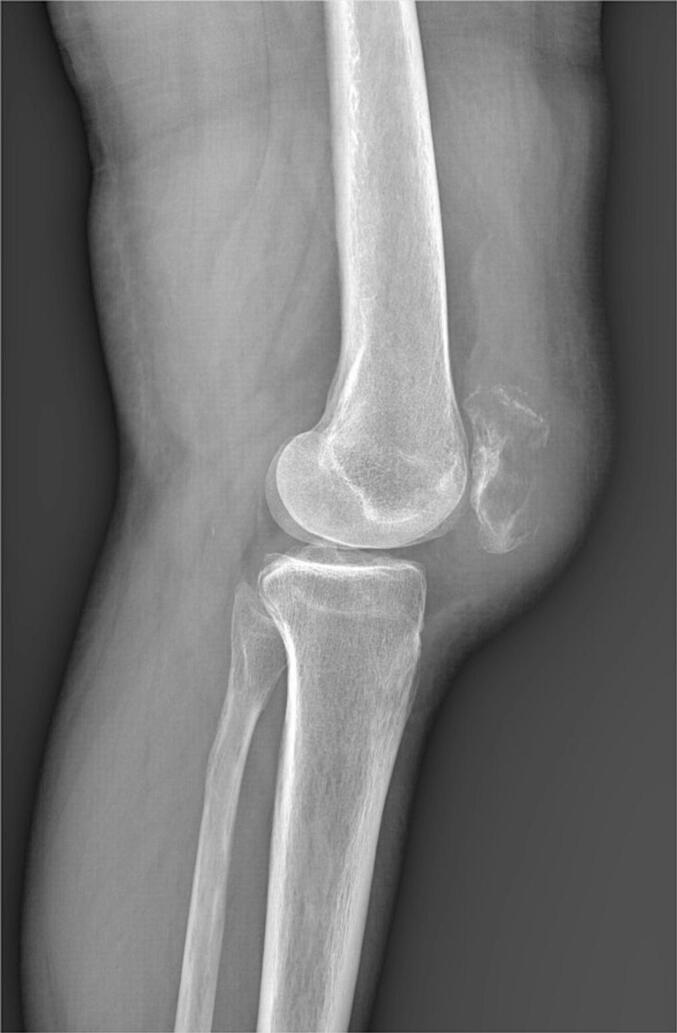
Conventional radiography:* Anteroposterior radiograph of the right knee showing a large, poorly defined lytic lesion involving the majority of the patella with cortical thinning.
